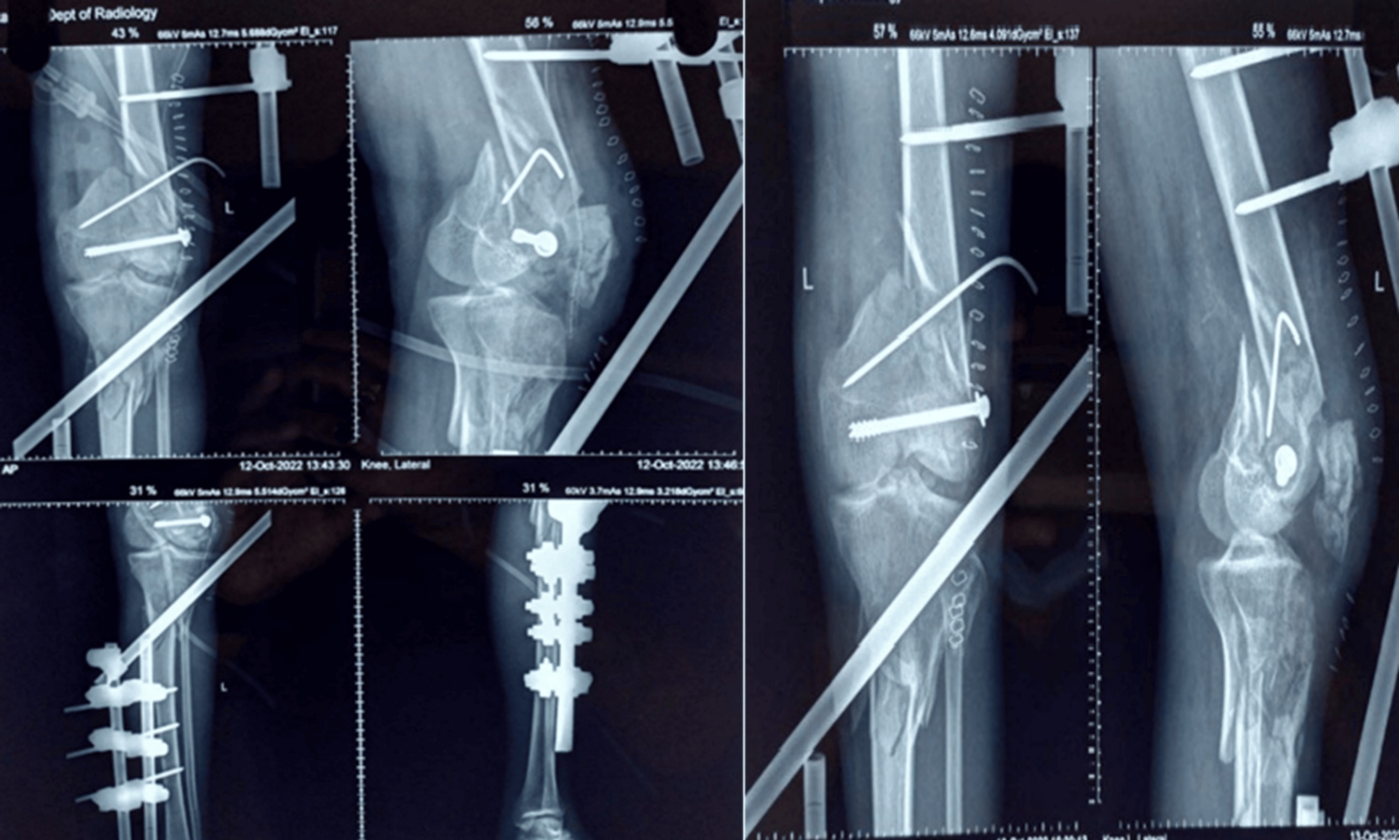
Postoperative radiograph showing a trans knee fixator with cortico-cancellous screw and K-wire in the distal femur in type IIc floating knee injury (open)
